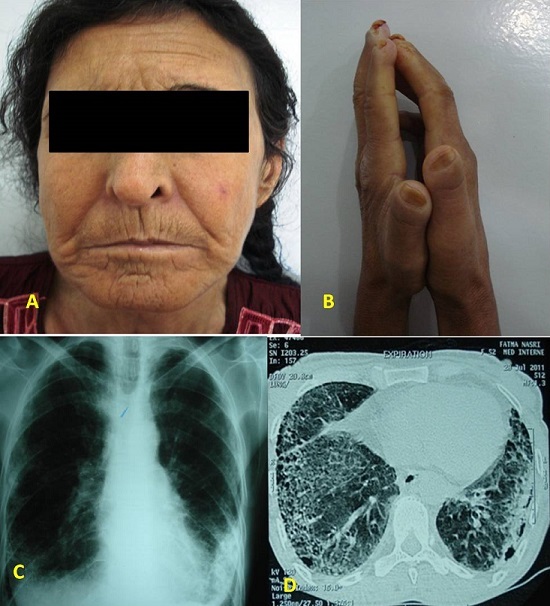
A) aspect de visage sclérodermiforme; B) sclérodactylie avec signe de la « prière » positif; C) radiographie de face objectivant le syndrome interstitiel pulmonaire; D) aspect de fibrose pulmonaire diffuse à la tomodensitométrie pulmonaire
